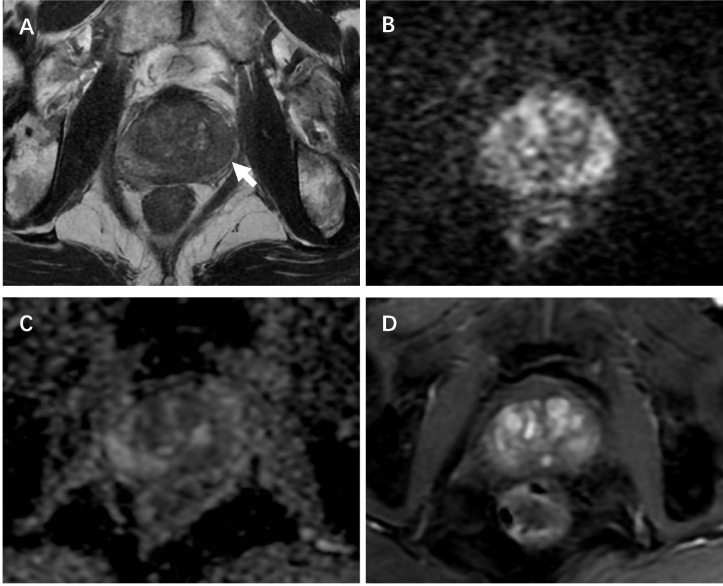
A 68-year-old man with PSA 22.4 ng/ml, were found a lesion (white arrow) in the left peripheral zone on (a) axial T2-weighted image, (b) axial diffusion-weighted image with b value of 2000 mm2/sec, (c) axial apparent diffusion coefficient map, and (d) axial dynamic contrast-enhanced image in prostate MRI. The PI-RADS scores assigned by the four readers were 2, 3, 3, and 3 in bpMRI respectively, as well as 2, 2, 3, and 3 in mpMRI respectively. mpMRI, multiparametric MRI; bpMRI, biparametric MRI; PSA, prostate-specific antigen; PI-RADS, Prostate Imaging Reporting and Data System.
